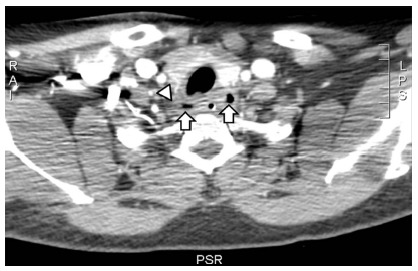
Cervical computed tomography (axial section, with contrasts, venous phase) emphasizing emphysema (long arrows) and perilesional inflammatory process (head of arrow)
